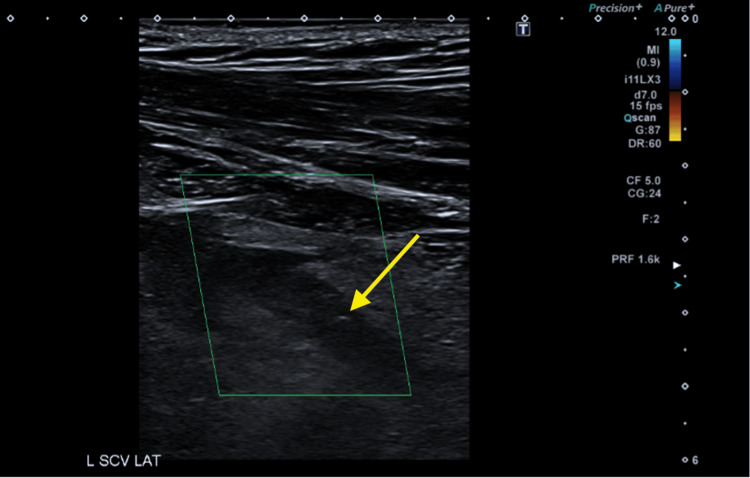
US shows echogenicity (yellow arrow) and lack of compression of the left subclavian vein (green box) US: ultrasound
